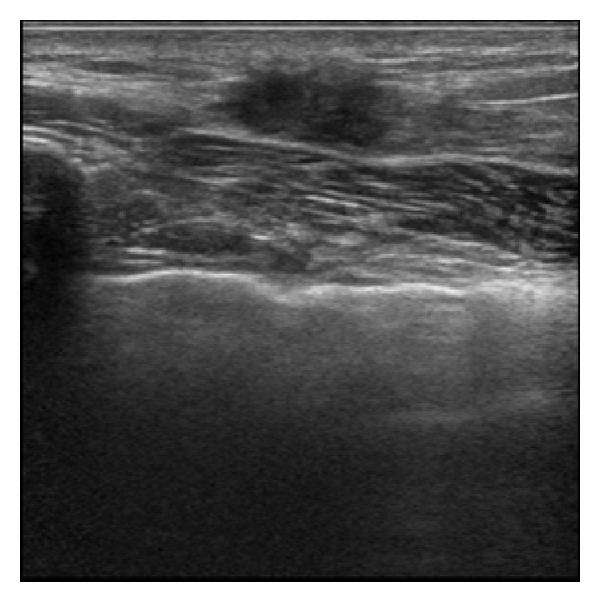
Ultrasonography revealed a hypoechoic lesion with an irregular margin and no acoustic shadow. The mass extended from the anterior border of the mammary gland.
